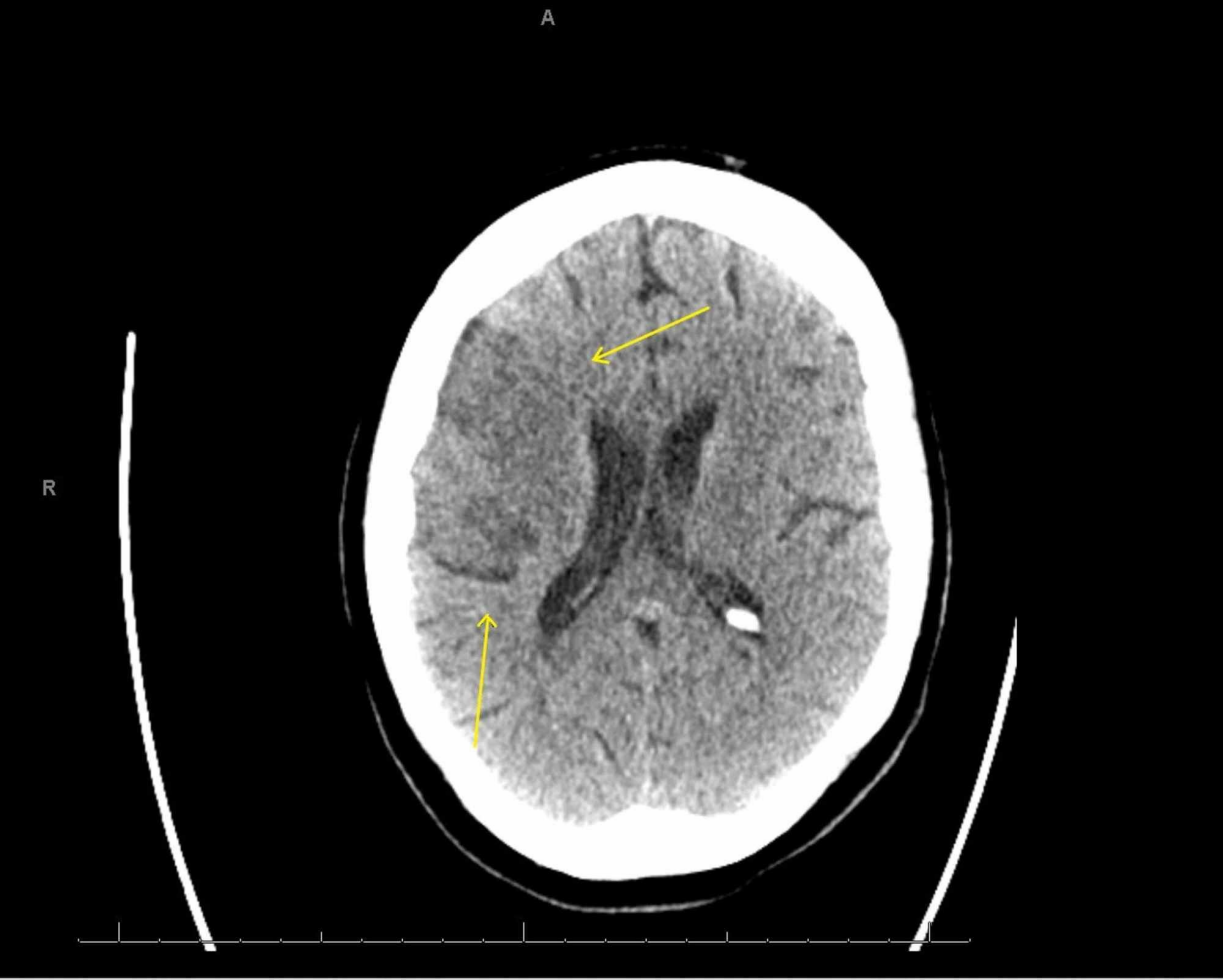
Non-contrast computed tomography (CT) of the head eight days after symptom onset significant for a new hypodensity in the right middle cerebral artery (MCA) distribution (arrows)
